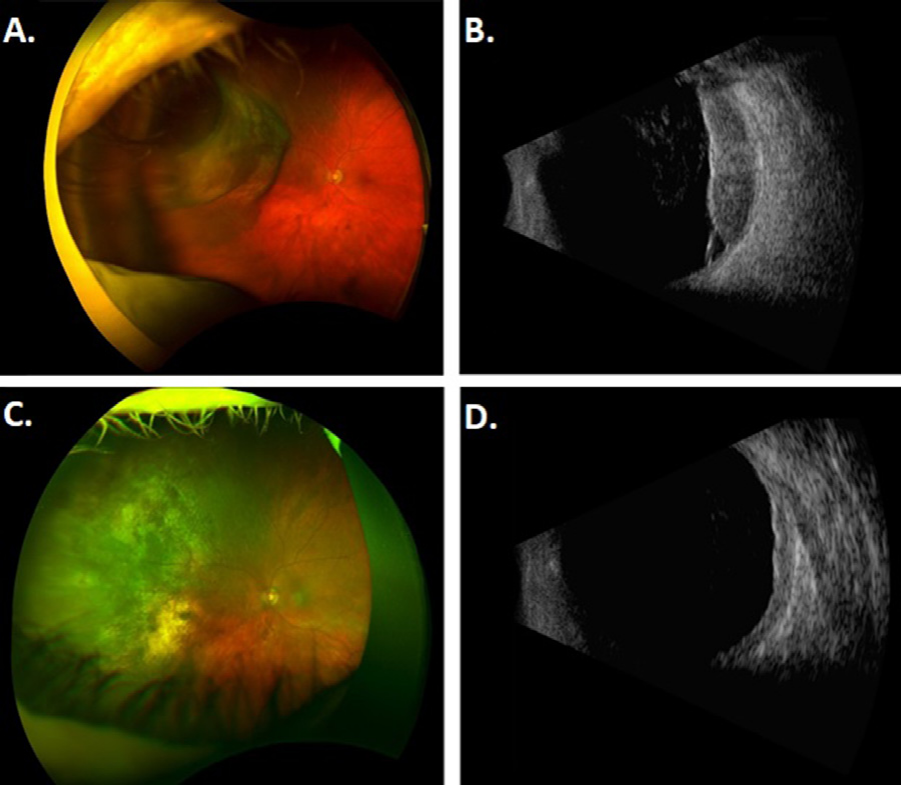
Tumor of patient B. (A) Fundoscopy at presentation. (B) Ultrasonography at presentation. (C) Fundoscopy at last follow-up. (D) Ultrasonography at last follow-up.
